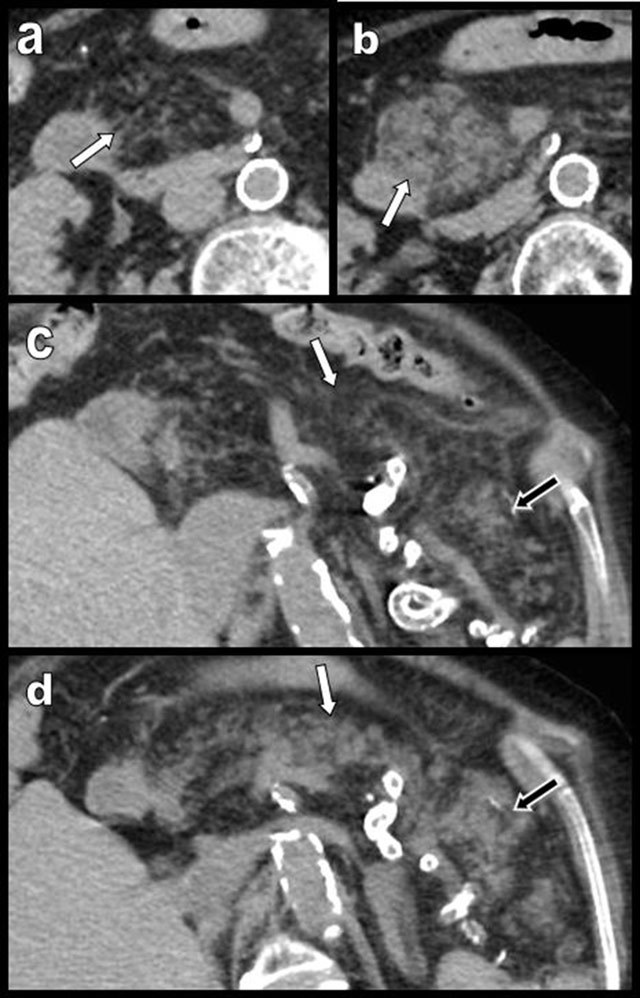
A 60-year-old woman presented with clinical and biological acute episode of pancreatitis. Unenhanced abdominal CT showed a moderately lipomatous but nearly normal cephalic (b) and corporeo caudal (d) pancreatic gland. However, on a previous CT found in the database, the pancreatic gland appeared extremely lipomatous (type 2b) (a and c). Due to edema, acute pancreatitis was responsible of the very unusual “reappearance” of the gland. Acute pancreatitis on a very lipomatous pancreas may mimic a normal pancreas.
